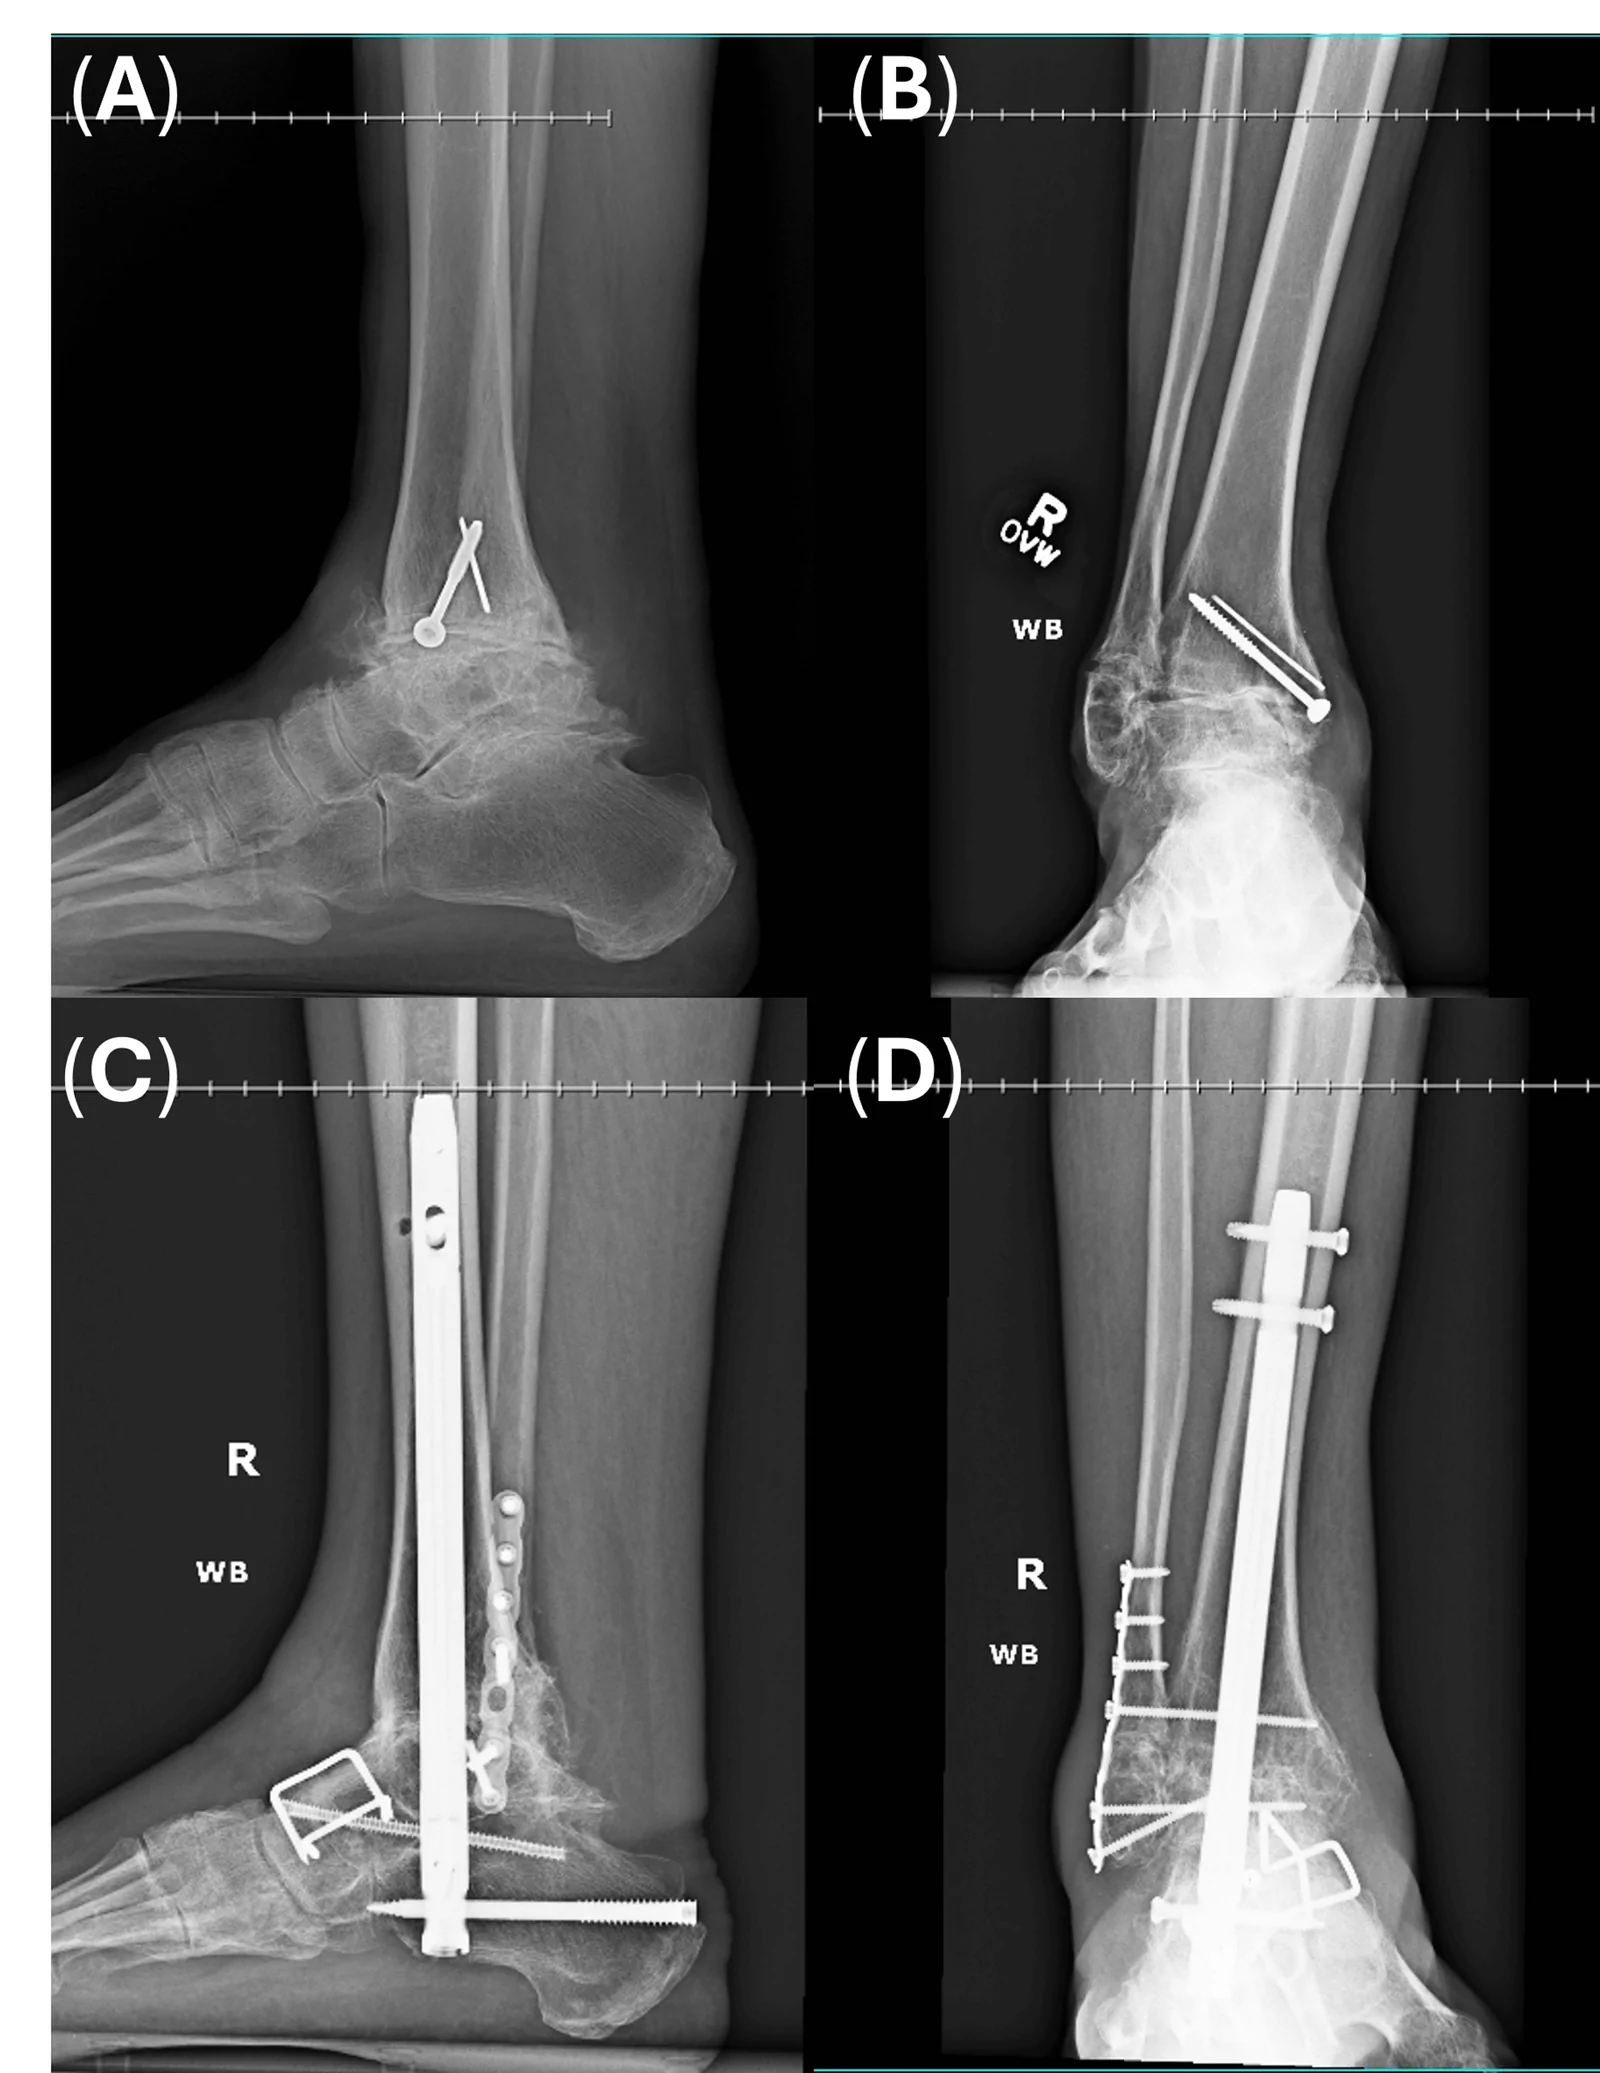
Example radiographic imaging from an ankle revision procedure (A, B) Baseline and (C, D) 20-week follow-up radiographs depicting successful fusion of a 62-year-old male participant who underwent removal of deep implanted hardware at the right ankle, a pantalar fusion, and repair of a fibular fracture non-union. Image courtesy: Dr. Kurt J. Hofmann
